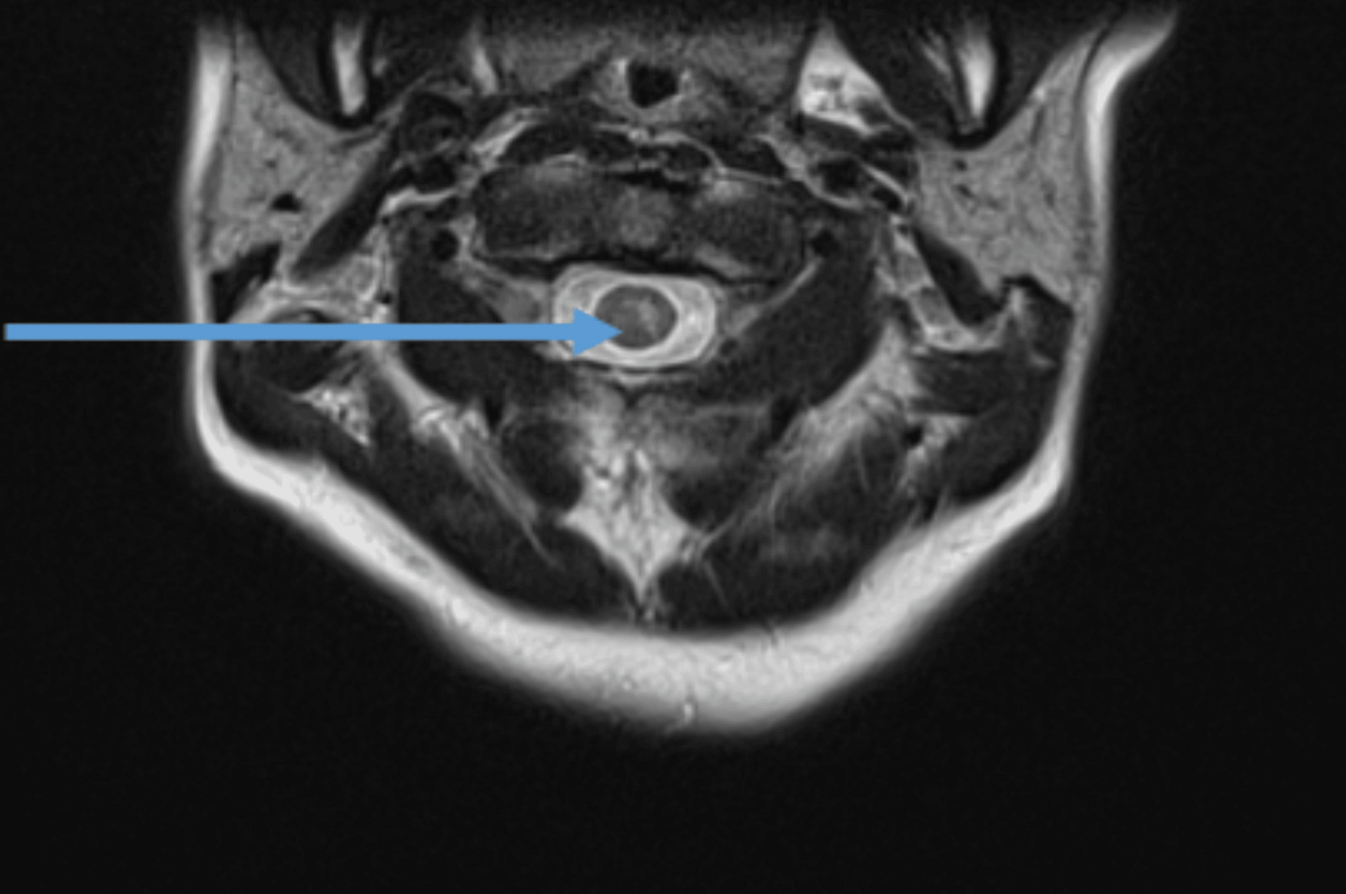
MRI brain: areas of altered signal-T2 hyperintensity with possible restricted diffusion in the brainstem and cervicomedullary junction, along the floor of the fourth ventricle MRI: magnetic resonance imaging
